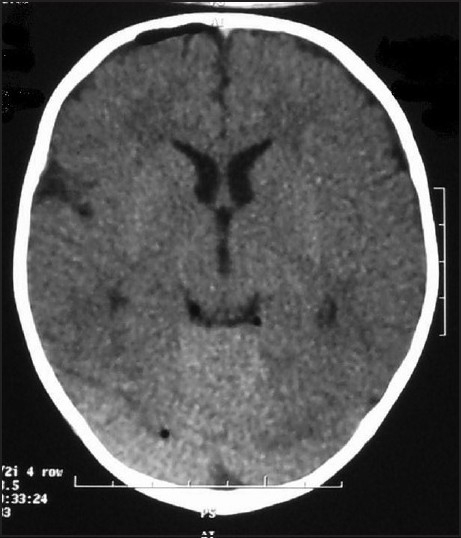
Brain computed tomography scan of the second patient shows a small rim of pneumocephalus
